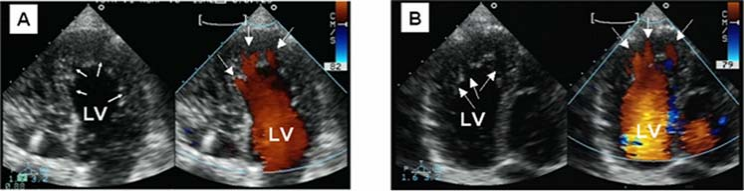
Imaging of NVM in the index patient (III:4, panel A) and her sister's daughter (IV:13, panel B). Shown are two-dimensional echocardiographs (ventricular part of a four-chamber view) in diastole, with (right) and without (left) color flow imaging. Arrows point to recesses in the myocardial wall.
